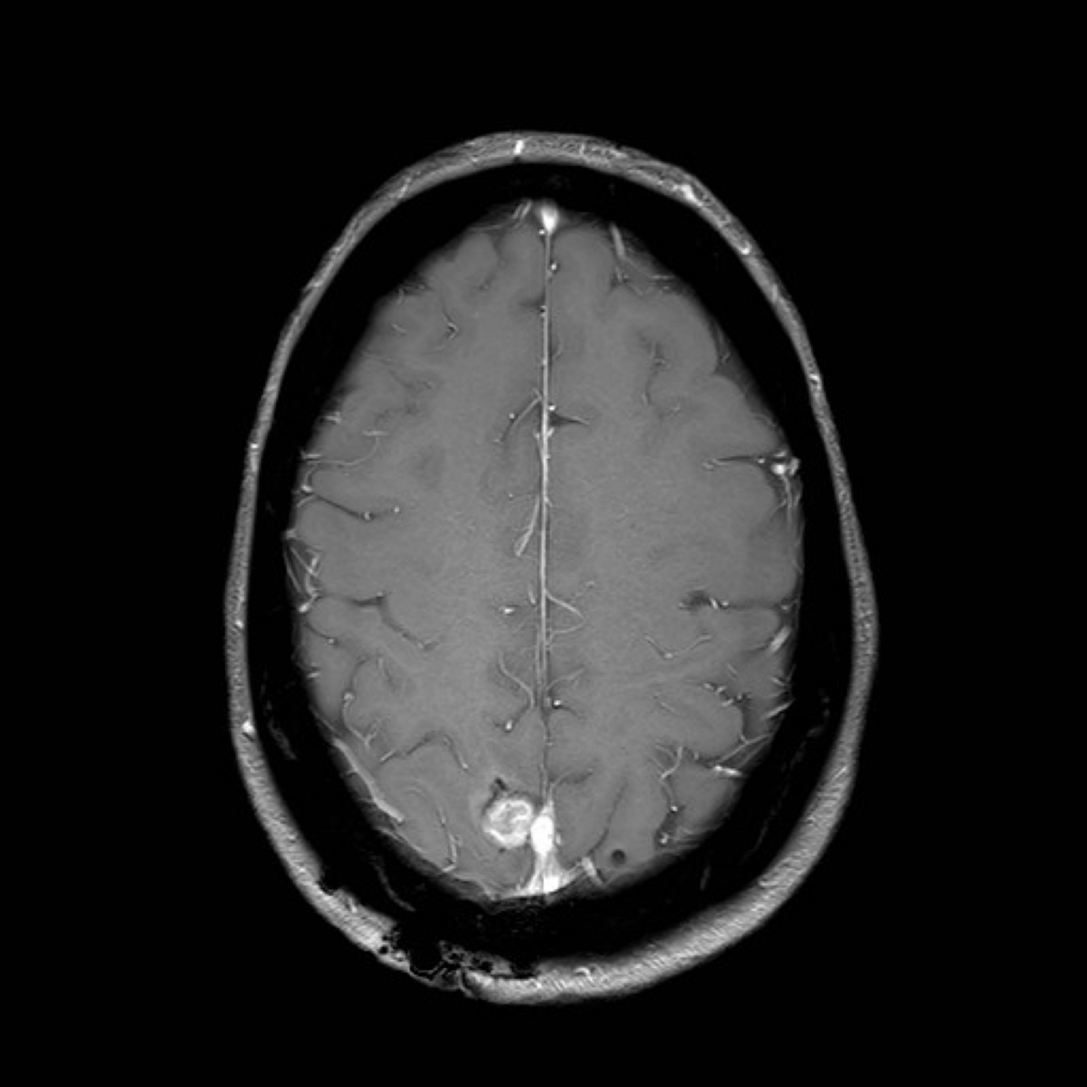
Axial T1 MRI FFE radiograph three months post-operation, showing a small nodular enhancement in the surgical cavity consistent with intraoperative observation of residual tumor MRI: magnetic resonance imaging; FFE: fast field echo
